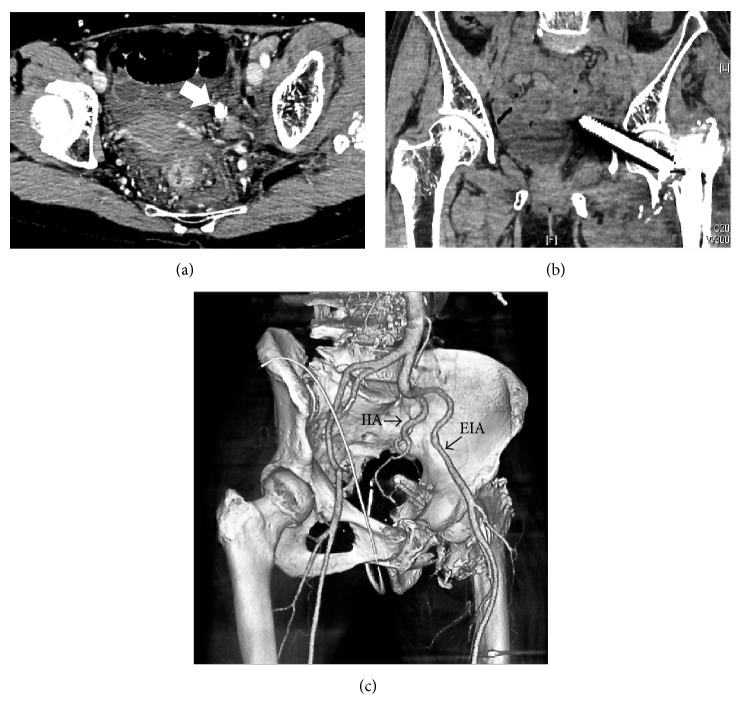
Contrast-enhanced computed tomography showing the lag screw located deep in the pelvis, between the internal and external iliac vessels, and tangent to the bladder and sigmoid. (a) Axial view (arrow: lag screw), (b) coronal view, and (c) reconstruction 3D image (EIA: external iliac artery, IIA: internal iliac artery).
